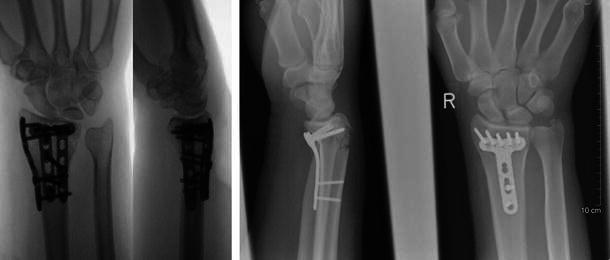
Postoperative x-rays of a dorsal (AO 23 C3) (*left*) and volar locking plate osteosynthesis (AO 23 A3) (*right*)
